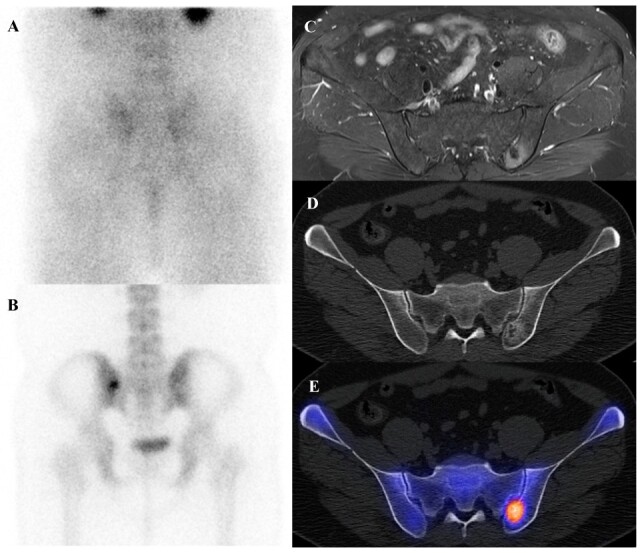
A 38-year-old male with osteoblastoma of the left iliac bone. (A,B) Bone scintigraphy (posterior view) shows no abnormal perfusion on the blood pool phase, but focal increased uptake in the left iliac bone on the delayed phase. (C) T2-weighted fat-suppressed magnetic resonance imaging demonstrates an irregular sclerotic lesion with surrounding bone marrow edema at the left iliac crest near the sacroiliac joint. (D,E) Bone single-photon emission tomography/computed tomography reveals an osteolytic lesion with a sclerotic rim and focal increased tracer uptake.
